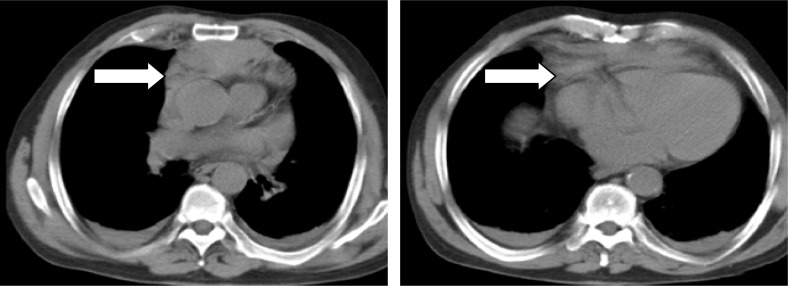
Chest CT scan of the patient after the administration of thrombolytic therapy indicating the retrosternal mass
